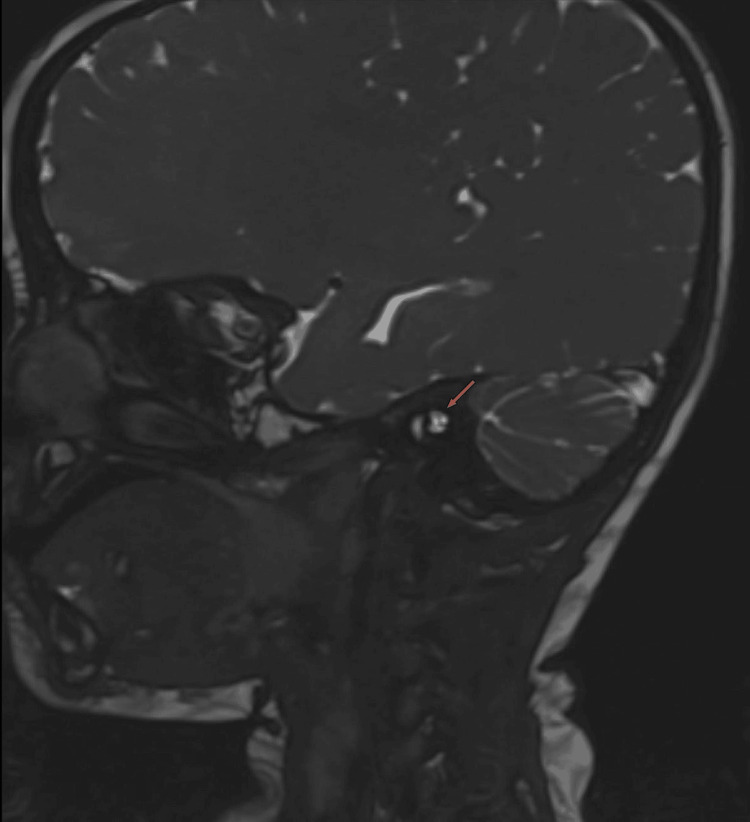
Magnetic resonance image of the right temporal bone Facial nerve and vestibular nerves seen within the right internal auditory canal (arrow) appear normal. However, cochlear division of the vestibulocochlear nerve is not visualized in the antero-inferior quadrant.
